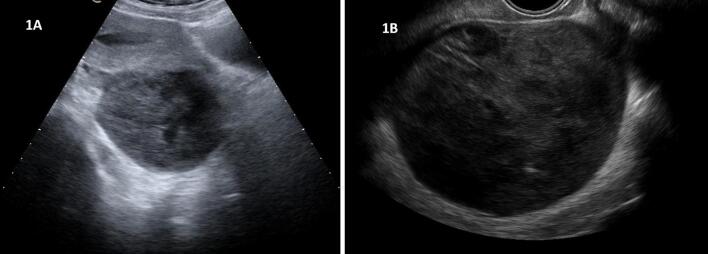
Radiological findings. (A) Transabdominal ultrasound: a large, complex left adnexal mass with heterogeneous echoes, mimicking an endometrioma. (B) Transvaginal ultrasound: Detailed view of the 12 × 10 × 7 cm mass oriented toward the left mesovarian region.
